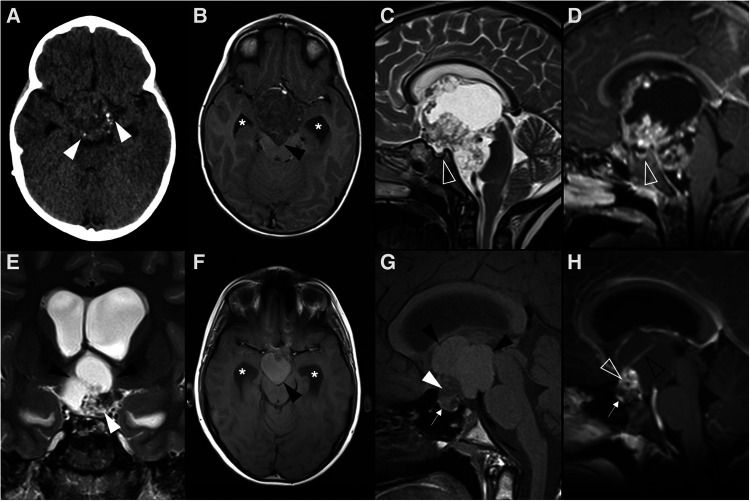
Adamantinomatous craniopharyngioma in 6-yearl-old boy (A–D) presenting with headaches. Axial head CT scan (A) shows an iso-hypodense expansile suprasellar lesion with multiple foci of calcification in the periphery (white arrowheads). Brain MR including axial T1WI (B), sagittal T2WI (C) and sagittal T1WI after gadolinium injection (D) shows a very large expansile lesion with heterogeneous signal intensity and enhancement, causing obliteration of the III ventricle and presenting extension towards the interpeduncular (black arrowhead) and pre-pontine cisterns with associated mass effect over the optic chiasm and optic tracts as well as brainstem. Note normal positioning and enhancement of the pituitary gland (empty white arrowhead) as well as moderate hydrocephalus (*). Adamantinomatous craniopharyngioma in a 13-year-old (E–H) boy presenting with headaches. Brain MRI including coronal T2WI (E) as well as axial (F) and sagittal (G) T1WI reveal a multilobular lesion centered in the suprasellar cistern and extending towards the interpeduncular cistern, separated from the pituitary gland, showing heterogenous signal intensity, including an inferior solid component (white arrowheads) and multiple cysts with spontaneous hyperintensity on T1 and T2 (black arrowheads). Post-contrast sagittal T1WI (H) reveals nodular enhancement of the solid component (empty white arrowhead) as well as peripheral enhancement of the cysts (empty black arrowhead). There is associated mass effect over the III ventricle, optic chiasm and tracts as well as moderate hydrocephalus (*).
